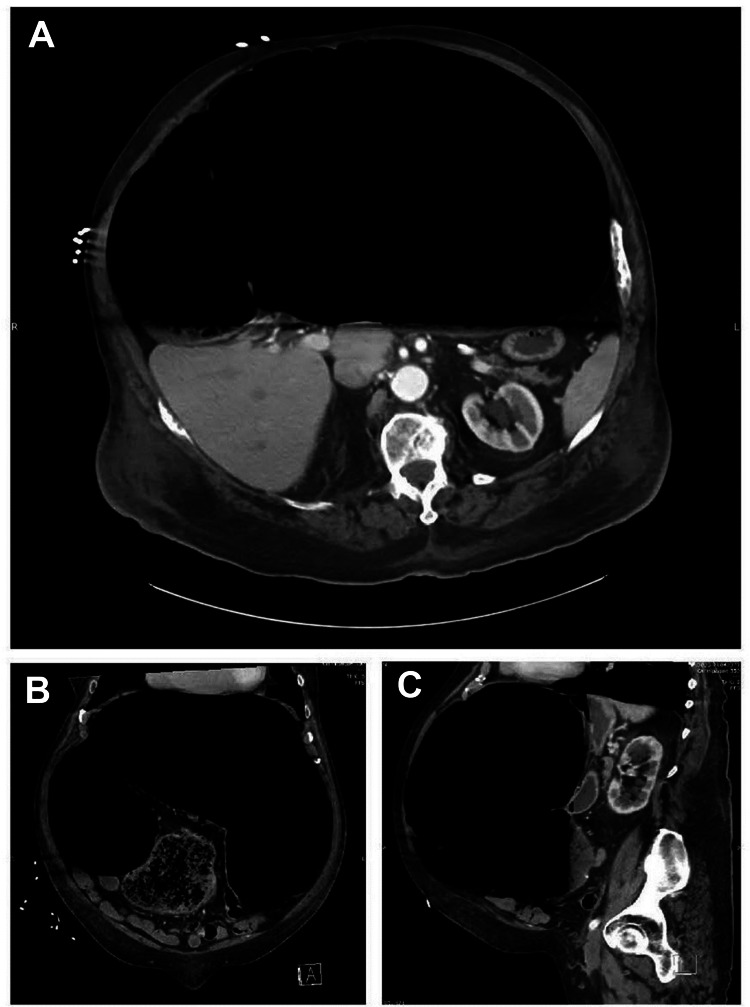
Non-contrast computed tomography of the abdomen and pelvis demonstrating massively dilated large bowel measuring up to 20 cm (A) Transverse view. (B) Coronal view. (C) Sagittal view.
